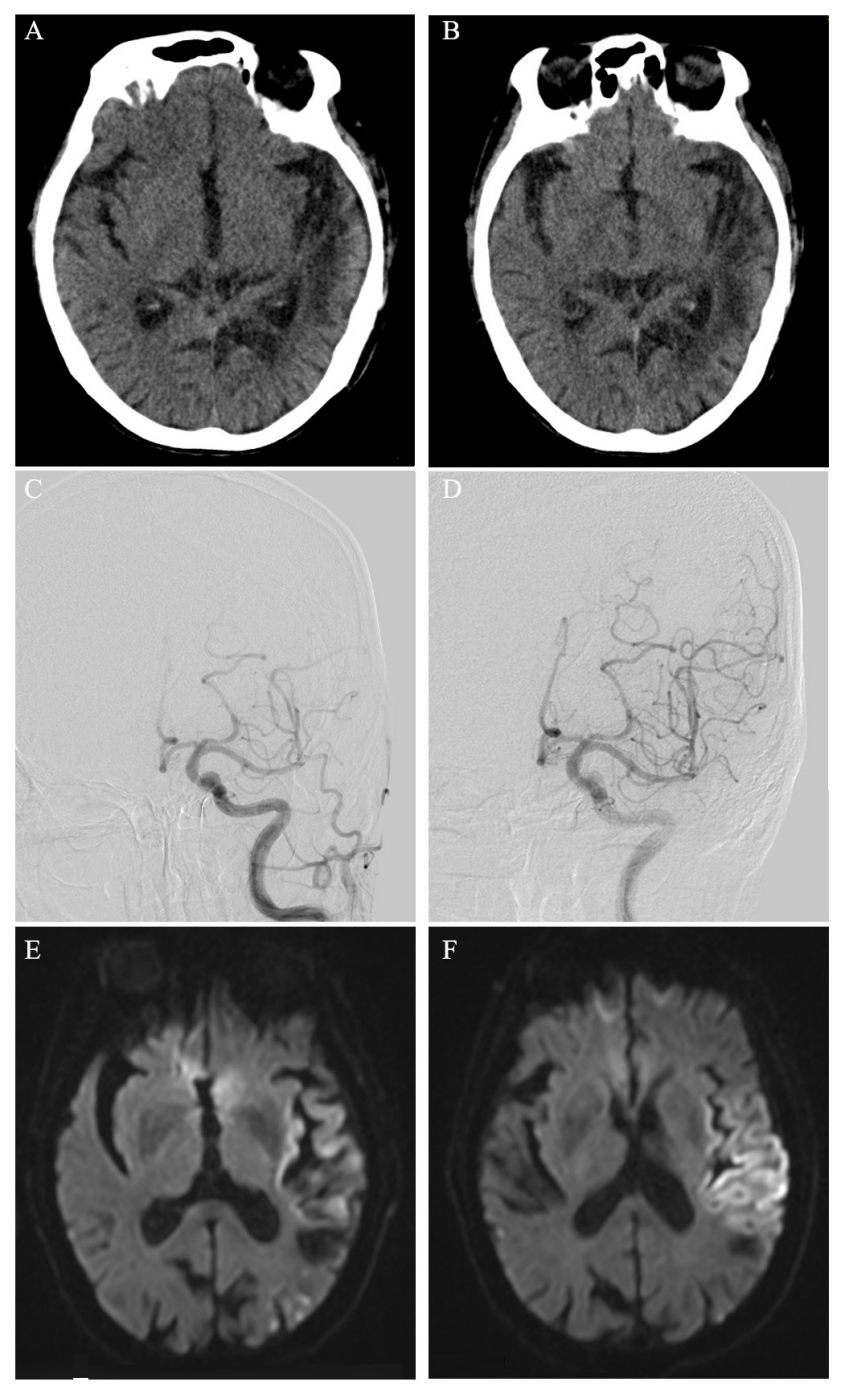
Head CT scan at admission (A), at the second day after endovascular treatment (B); DSA image of left internal carotid artery and the vascular branches before endovascular treatment (C) and after endovascular treatment (D); Brain DWI image after endovascular treatment (E, F).
